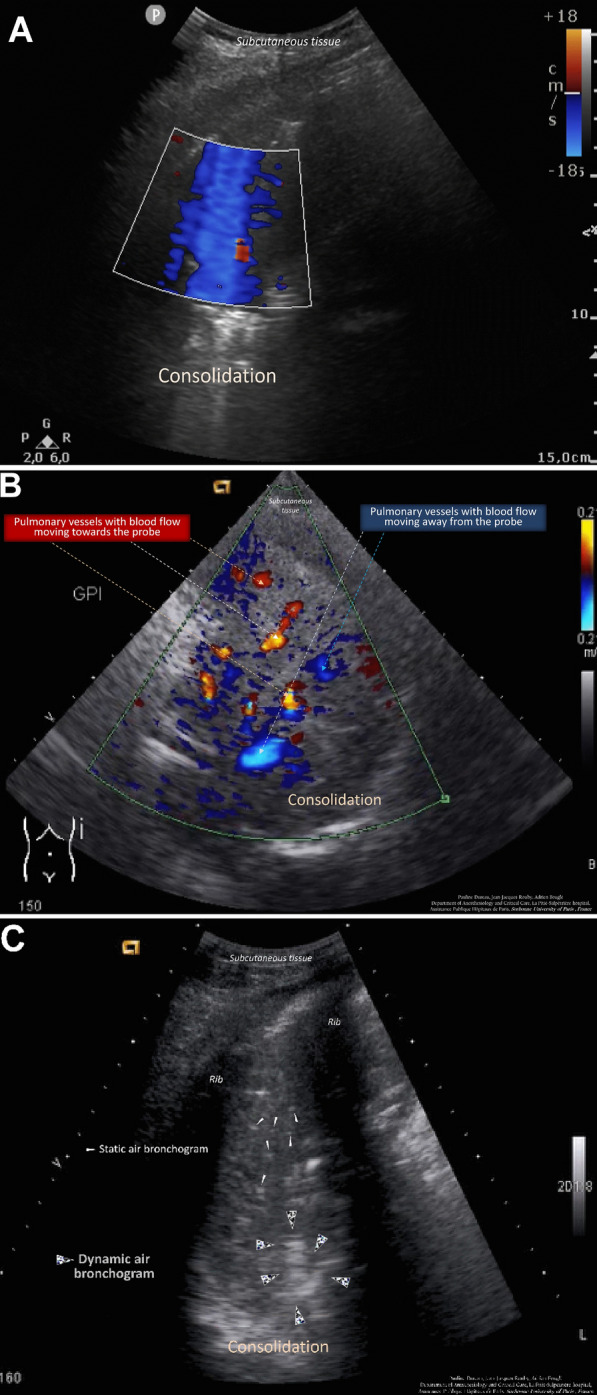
A Color Doppler lung ultrasound in the consolidated left lower lobe in a 54-year-old patient without pneumonia on venoarterial extracorporeal membrane oxygenation. The extracorporeal membrane oxygenation was initiated 2 days before and pneumonia was ruled out by a bronchoalveolar lavage retrieving 2.10^2^ orophayngeal flora. Color signals are diffuse and changing resulting from interferences caused by respiratory movements and heart beats. Corresponding to Video S1A (Additional File 1). B Color Doppler intrapulmonary flow detected in the consolidated right lower lobe of a 43-year-old patient with pneumonia on venoarterial extracorporeal membrane oxygenation. The extracorporeal membrane oxygenation was initiated 12 days before for a cardiogenic shock and pneumonia was confirmed by a bronchoalveolar lavage retrieving 10^4^
